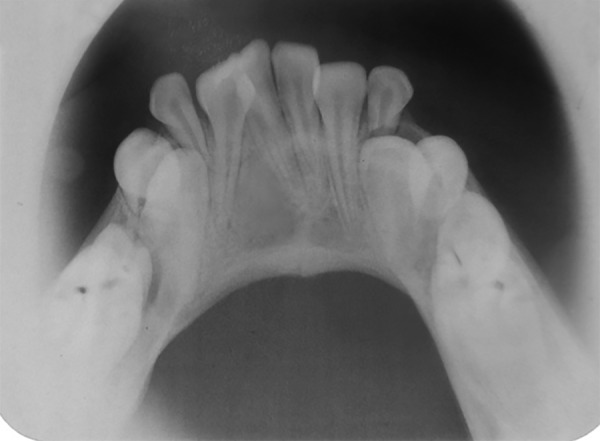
Mandibular occlusal view radiograph showing fusion of mandibular teeth with talon cusp with respect to 41 and 42
